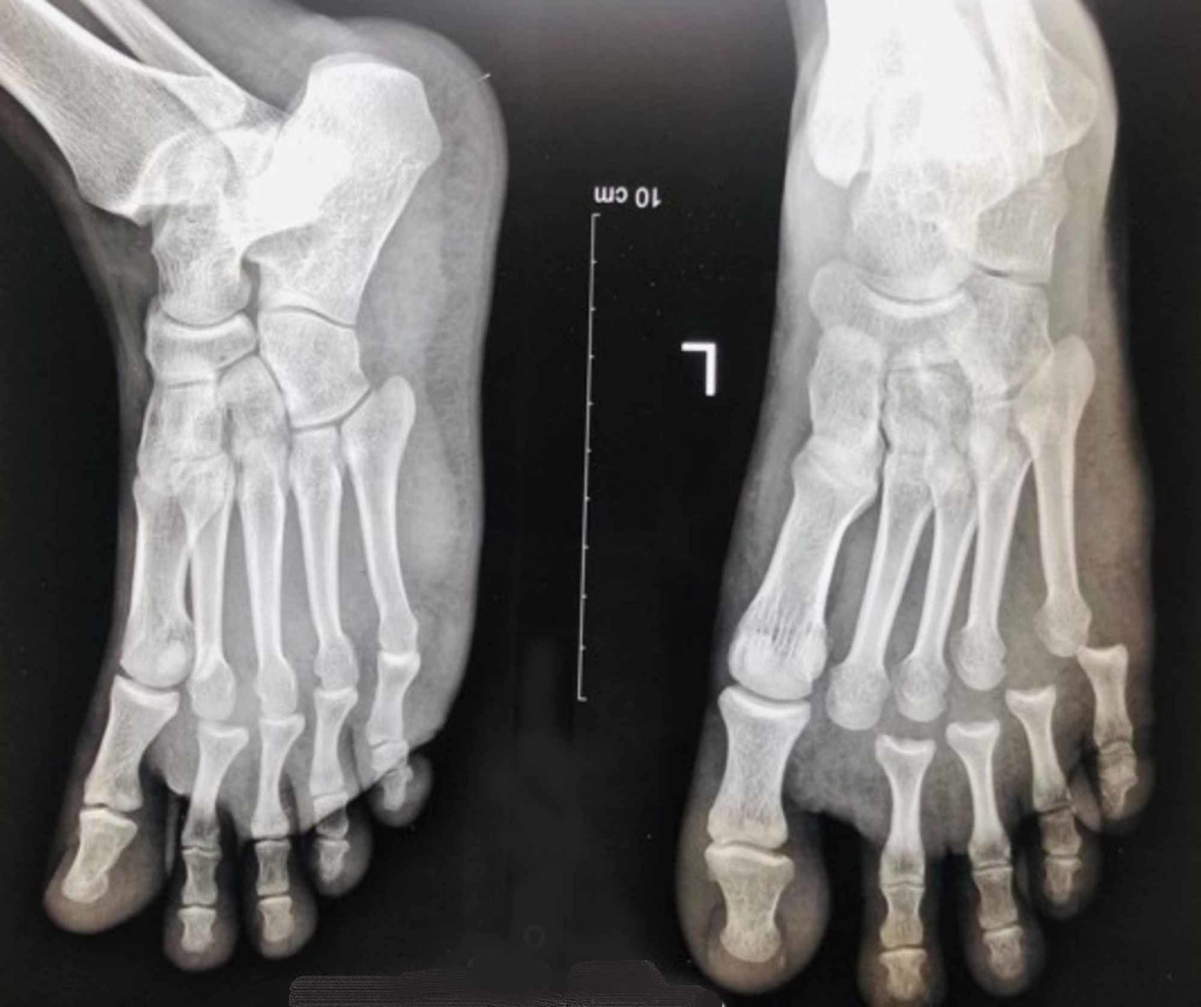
X-Ray of left foot showing dislocations of second to fifth metatarsophalangeal joints with no associated fractures.
